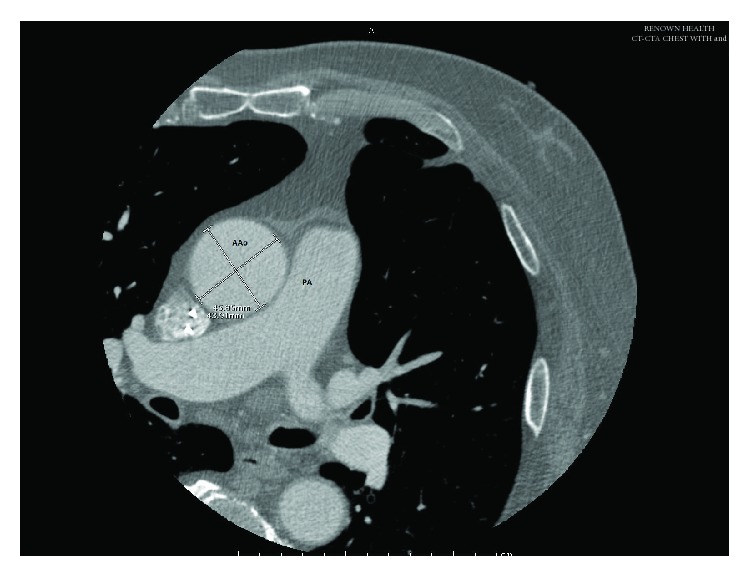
Computed tomography (CT) of the chest showing the enlarged ascending aorta measuring 4.6 × 4.3 cm. AAo, ascending aorta; PA, pulmonary artery.
